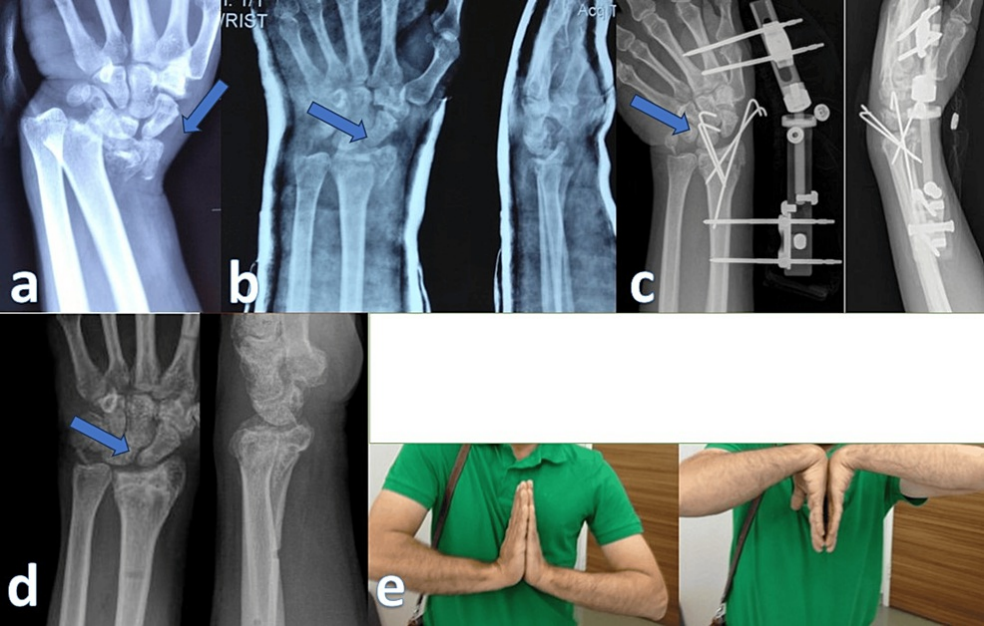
External fixation. (a) Intra-articular distal radius fracture due to high-energy injury before reduction. Arrows indicate the intra-articular fragment. (b) First, it was aimed to protect the neurovascular structures with closed reduction (control radiograph immediately after reduction). (c) However, due to joint stepping after reduction, the patient underwent joint reduction with external fixation and K-wires (three weeks after surgery). (d) Final radiograph at the 13th month (arrow indicates articular fragment). (e) Good clinical outcomes despite some metaphyseal collapse in the 13th month.
